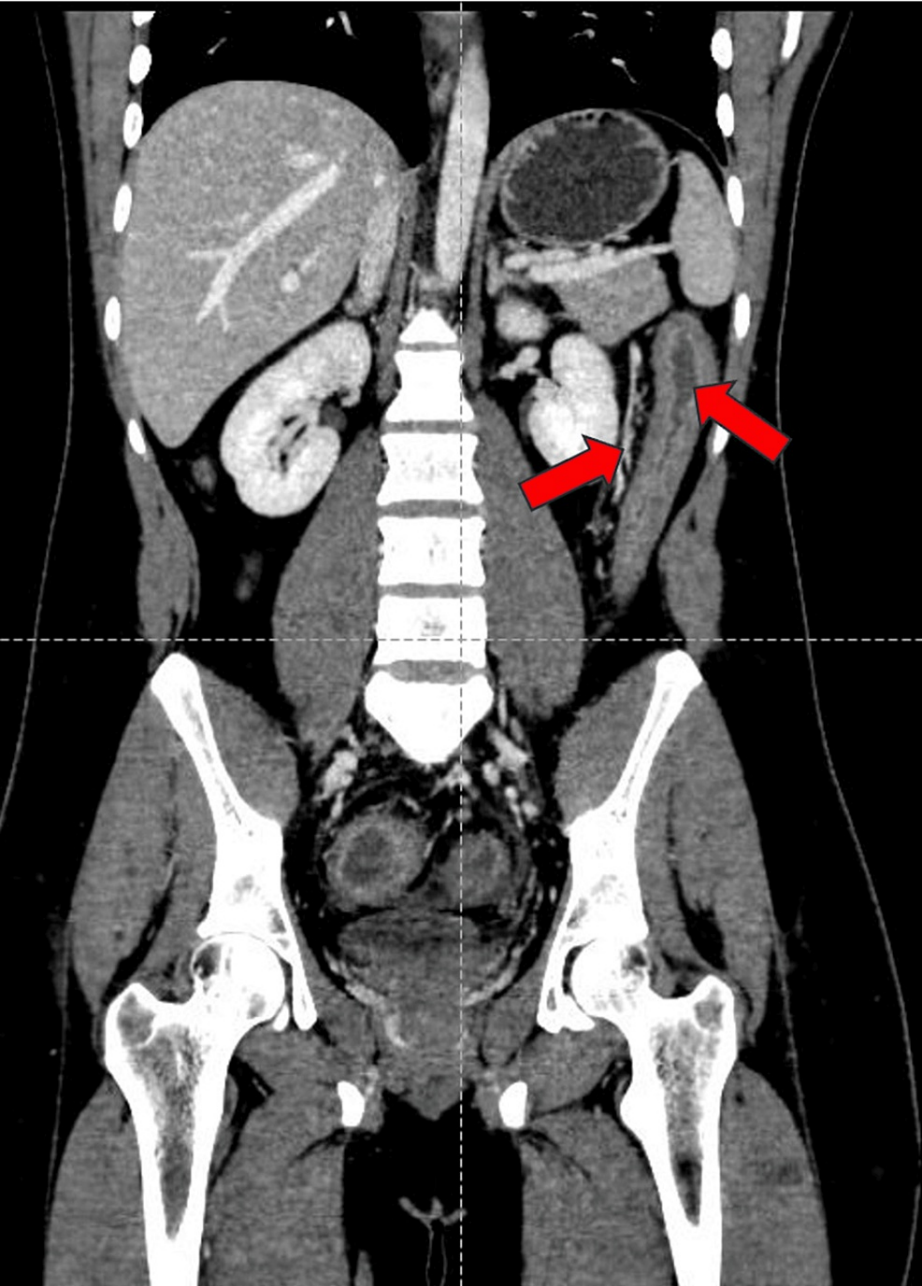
CECT-A/P coronal section: diffuse wall thickening involving the descending colon with serosal hyperemia (red arrows). CECT-A/P: contrast-enhanced computed tomography of the abdomen and pelvis
